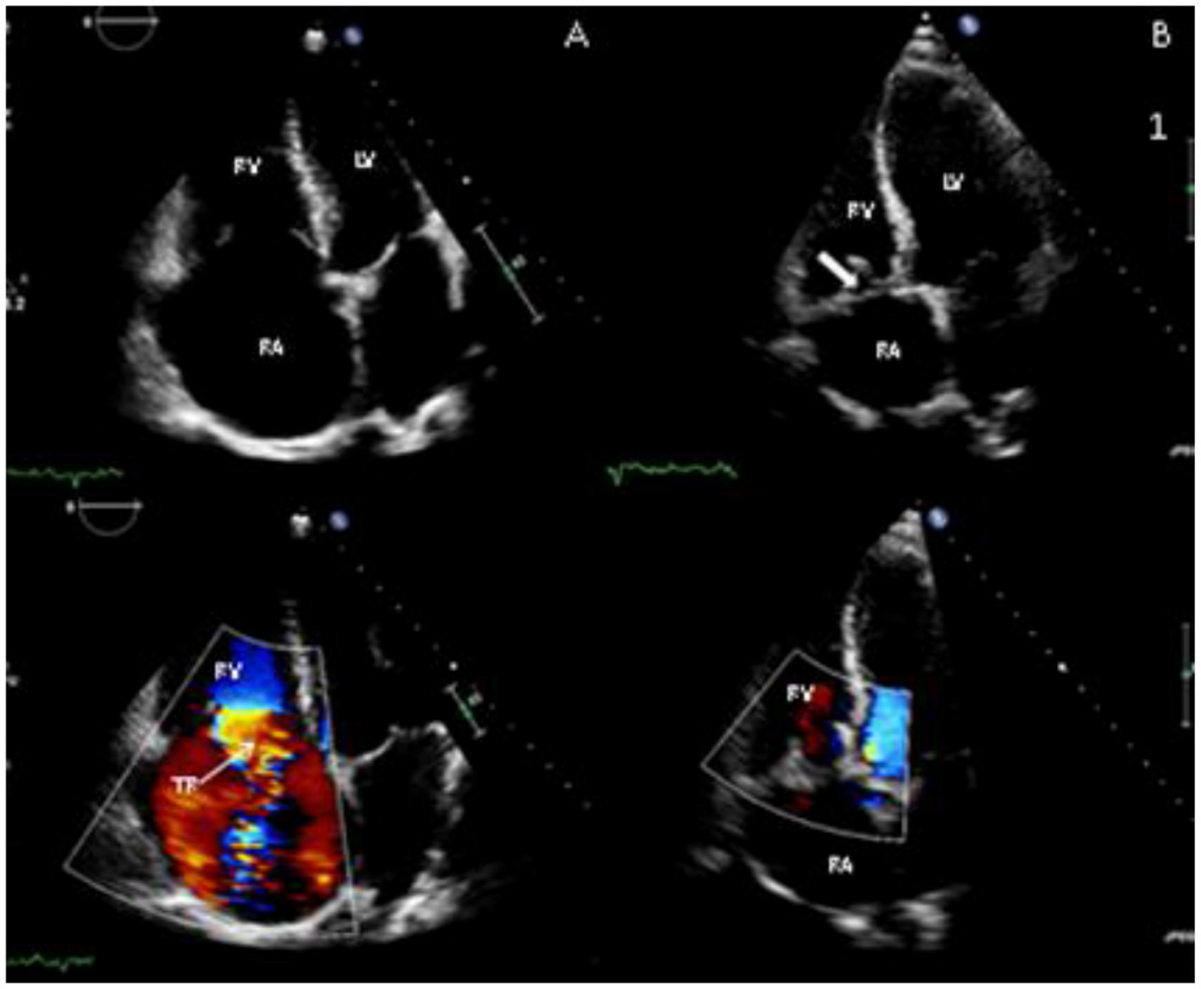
Transthoracic echocardiography images before surgery (A) and after surgery (B) showing apical 4-chamber view of the tricuspid valve (TV). In the pre-surgery images there is failure of leaflet co-aption (note closed position of mitral valve) due to valve degeneration, with severe tricuspid regurgitation (TR) on colour Doppler (lower panels); post-surgery, the right ventricle (RV) and right atrium (RA) have reduced in size and there is a tricuspid valve bioprosthesis (thick arrow) *in situ*, with resolution of TR.
